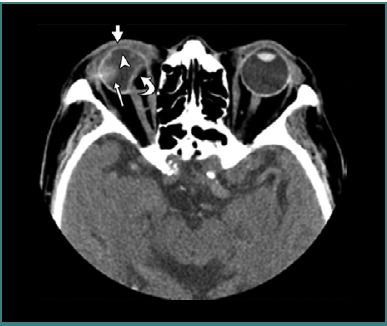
Axial computed tomography scan without contrast of a right open globe injury. The thick straight arrow indicates eyelid hematoma, the thin straight arrow represents vitreous hemorrhage, the arrowhead shows lens dislocation, and the curved arrow highlights scleral wall irregularities. Image reproduced with permission from the American Roentgen Ray Society: Yuan WH, Hsu HC, Lin YY, Liao SW. CT of globe rupture: analysis and frequency of findings. AJR Am J Roentgenol. 2014;202(5):4-5. ^©^ 2014 American Roentgen Ray Society.
